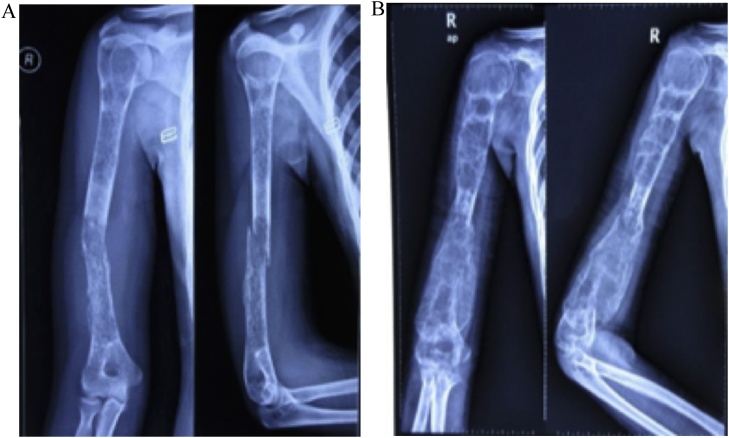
Anteroposterior and Lateral Radiograph of Humerus. A. Initial state, B. Post neoadjuvant chemotherapy.
